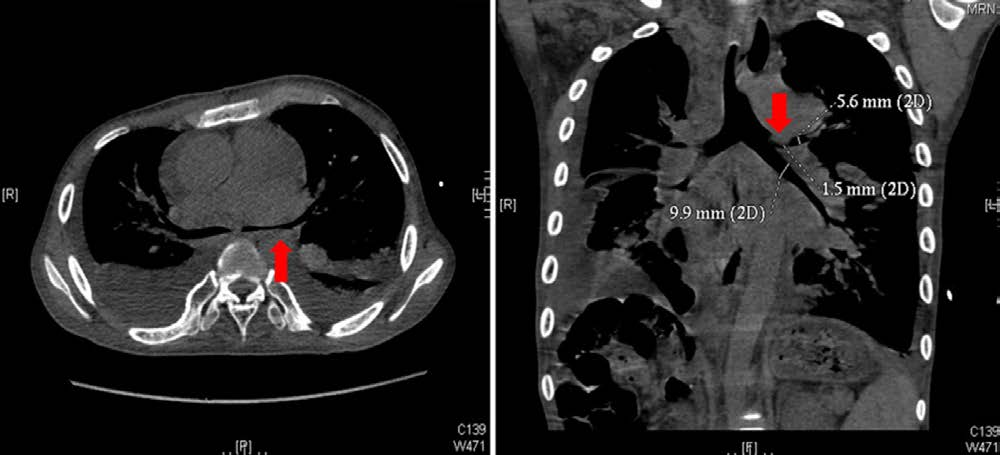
Axial mediastinal window CT (A) and coronal reconstructed CT (B) demonstrate narrowing of the anteroposterior diameter of the left main bronchus and the orifice of the left upper lobe bronchus (red arrows). CT = computed tomography.
